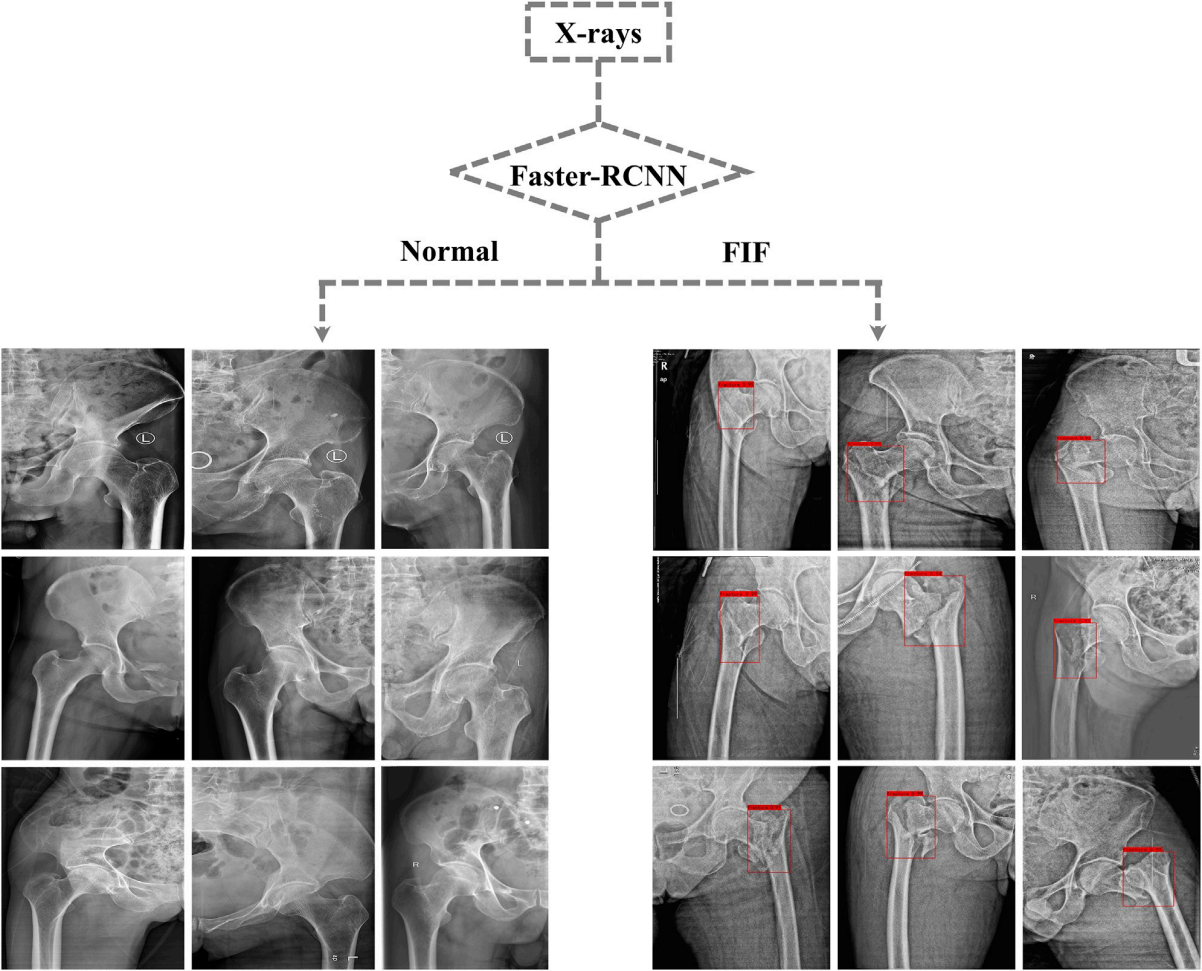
Part of output X-rays from the test dataset. Suspicious fractures were labeled with a red rectangle by the Faster-RCNN algorithm.
